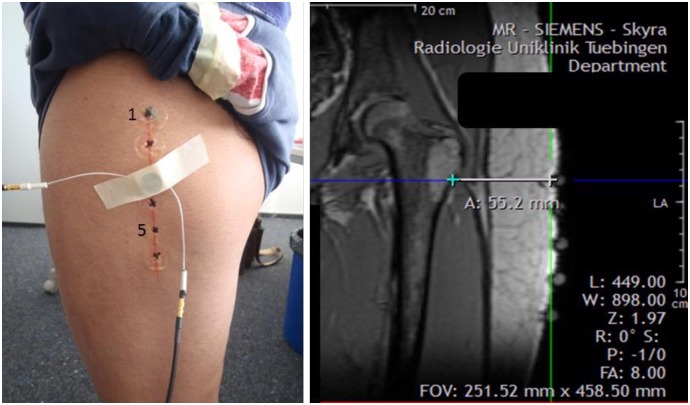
Ultrasound transducer placed on one of the marked positions on subject #1 (left). MRI of left limb of subject #1 with visible MRI markers (frontal plane) (right).
